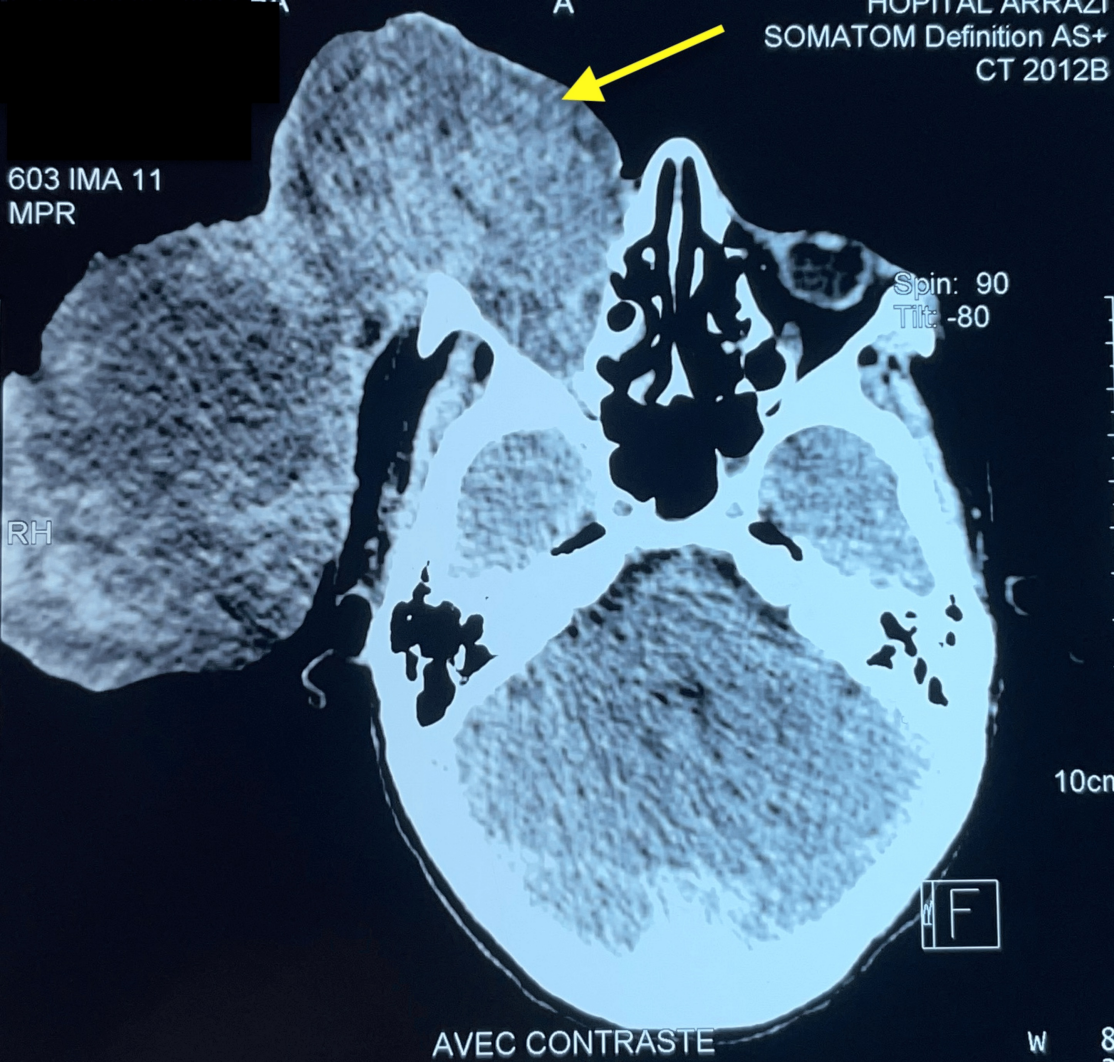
The orbital and cranial CT scan showed a large tumor process of the right eyeball measuring 18 x 12 x 14 cm, after chemotherapy (yellow arrow).
